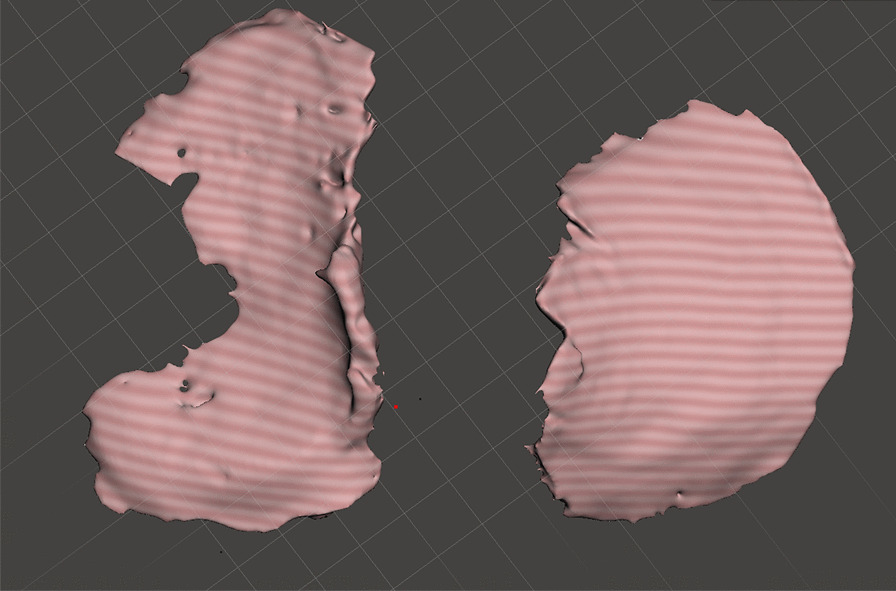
CT cartilage model showing lateral tibial plateau (left) and medial tibial plateau (right)
